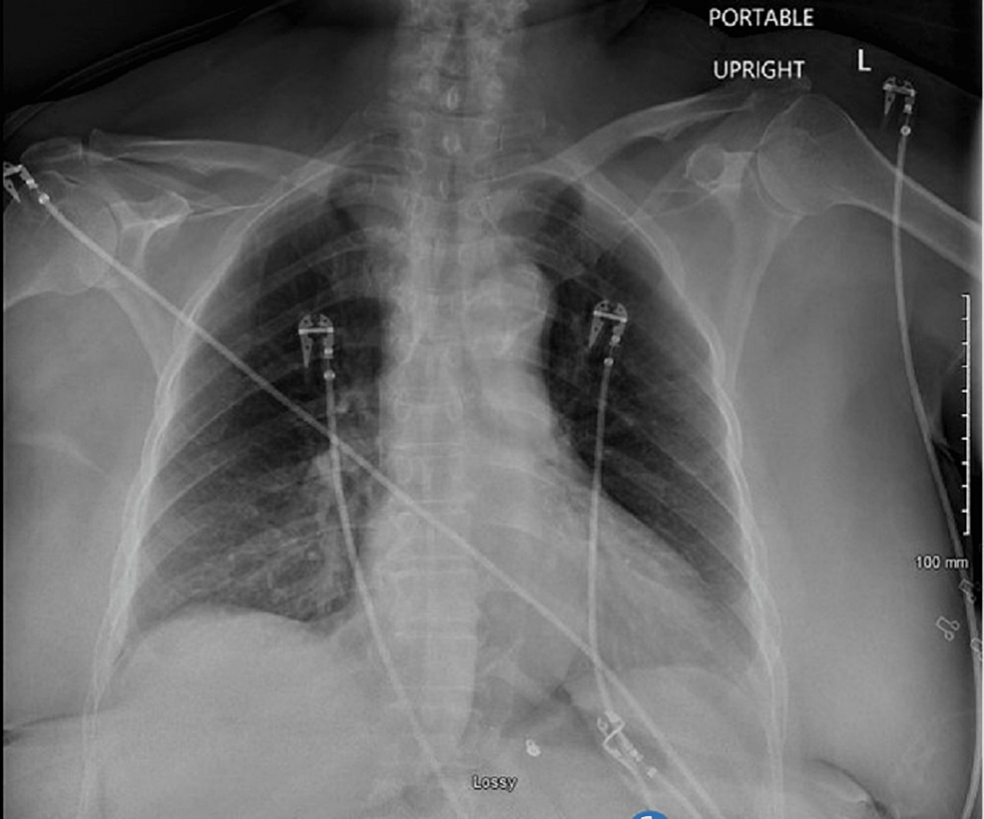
Initial chest radiograph scan showing no active signs of disease upon presentation
